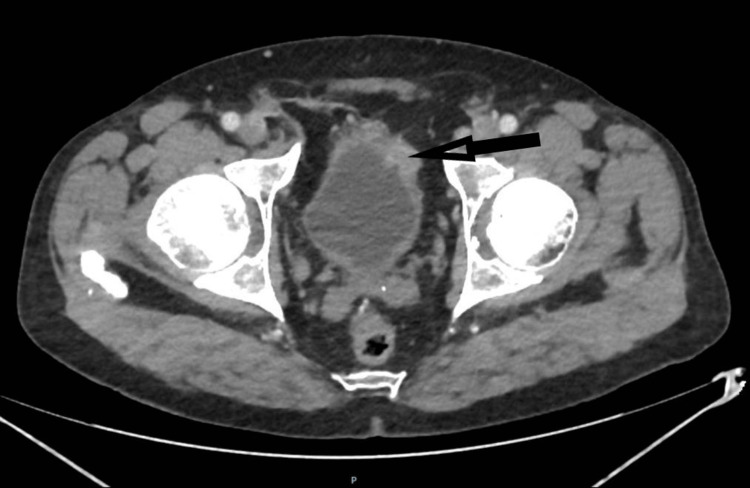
Computed tomography scan image showing a tumor on the anterior wall of the bladder
